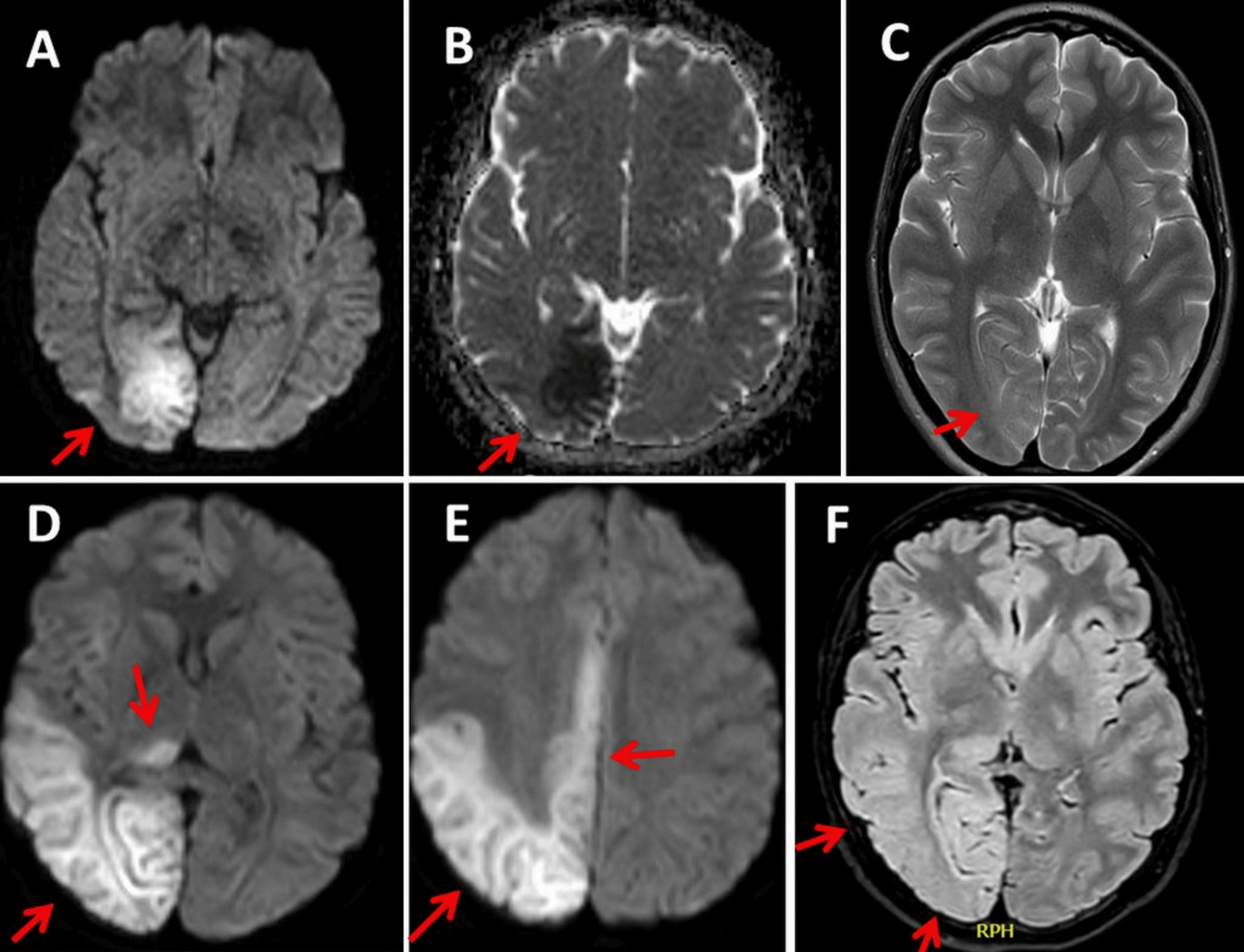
MRI of the brain at admission (Day 1) shows an occipital lesion that was hyperintense on T2/FLAIR (panel C) and DWI (panel A) and hypointense on ADC (panel B). The cerebral MRI on hd3 shows an enlargement of the right occipital lesion (panels D, E, F) FLAIR: fluid-attenuated inversion recovery; DWI: diffusion-weighted imaging; ADC: apparent diffusion coefficient
